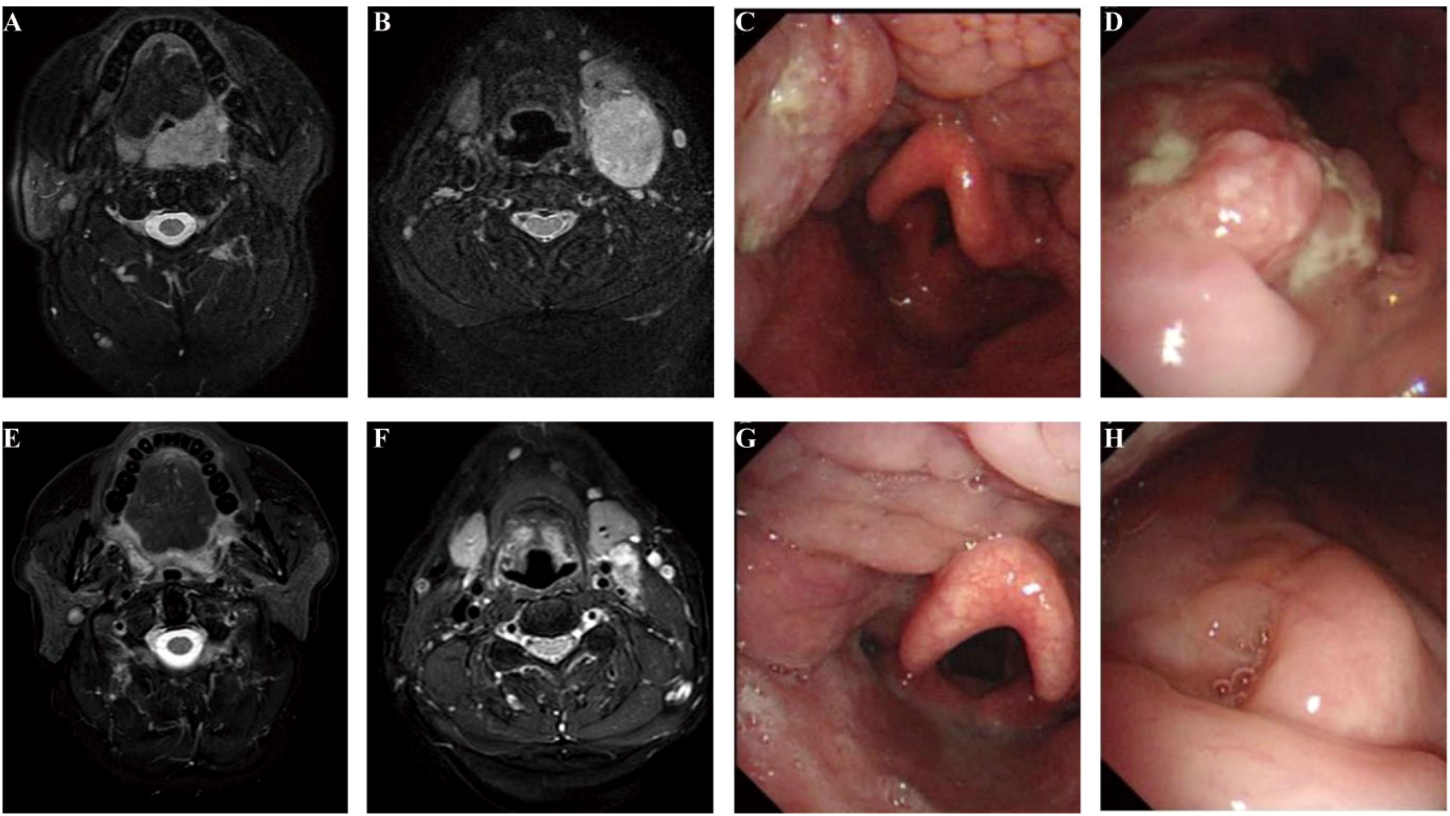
Accurate assessment of the response to neoadjuvant therapy remained challenging. Patient 5 baseline MRI (A, B), endoscopy (C, D), and examination after neoadjuvant treatment (E–H). The SLD change was only 37.3%, which was evaluated as PR according to RECIST v1.1, but the patient achieved pCR after the surgery. SLD, sum of the longest diameters; PR, partial response; RECIST, Response Evaluation Criteria in Solid Tumors; pCR, pathological complete response.
